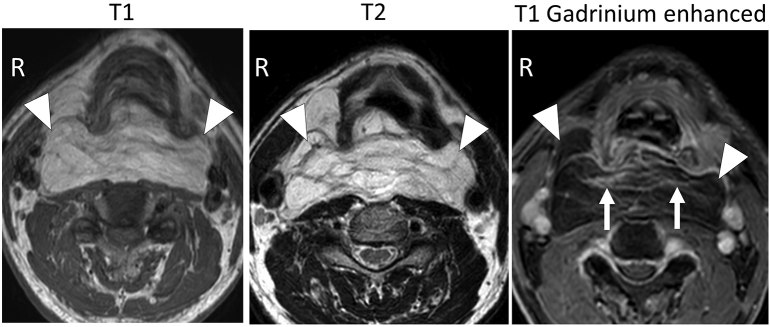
Magnetic resonance imaging. The tumor showed high intensity in T1 and T2 weighted images (arrowhead). Septum-like structures in the tumor were enhanced with Gadrinium (arrow).
